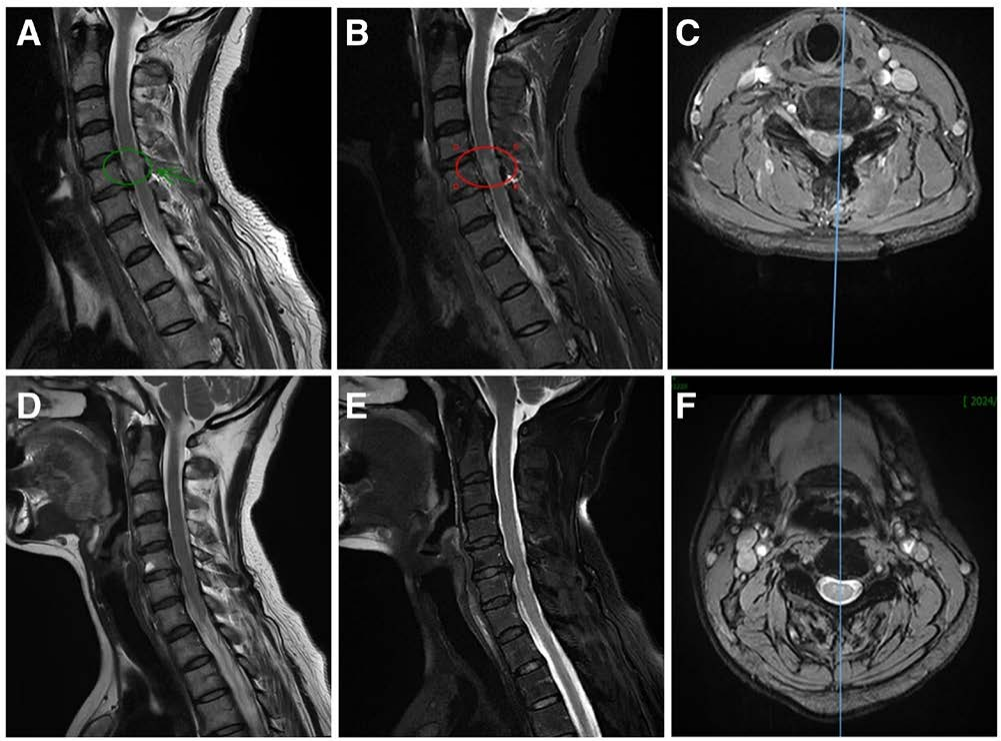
The patient underwent an MRI on the third postoperative day. Sagittal MRI images (A, T2W1; B, T2W1 STIR) revealed an abnormal T2 hyperintensity in the spinal cord at the cervical 5 vertebra level. The T2W1 STIR axial MRI (C) of the spinal cord revealed the aberrant signal bias to the left. MRI imaging at 6 months post-surgery. The T2 hyperintensity of the spinal cord at the level of the cervical 5 vertebrae was much lower than it had been before, as shown by sagittal MRI images (D, T2W1; E, T2W1 STIR) and axial MRI (F, T2W1 STIR). MRI = magnetic resonance imaging.
